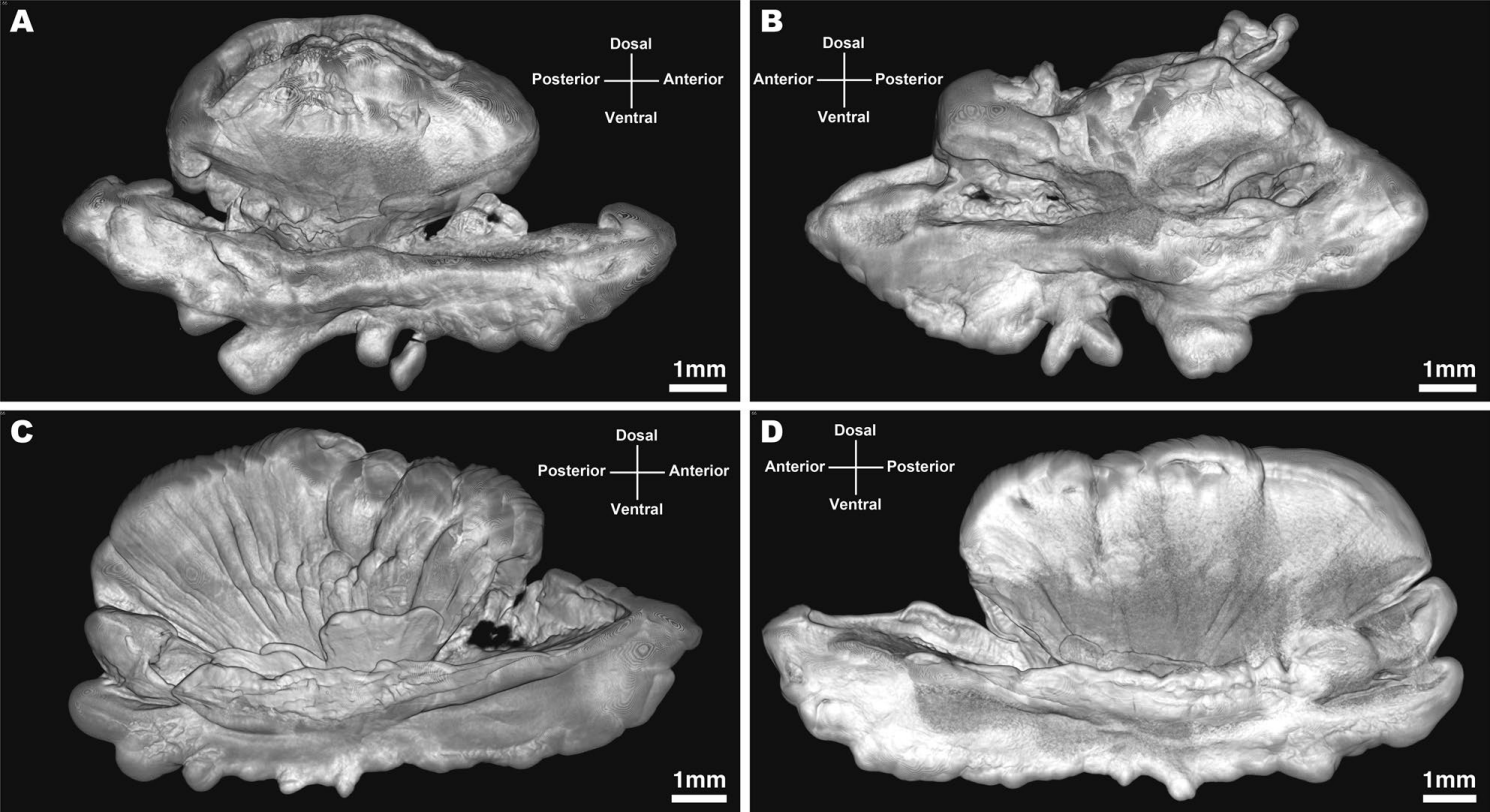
Narcetes shonanmaruae*. Micro-computed tomography images of otoliths. (a) and (b) Holotype (SH8-69). (c) and (d) Paratype 1 (SH8-43). (a) and (c) Medial view of left otolith. (b) and (d) Medial view of right otolith. Scale bar: 1 mm.
